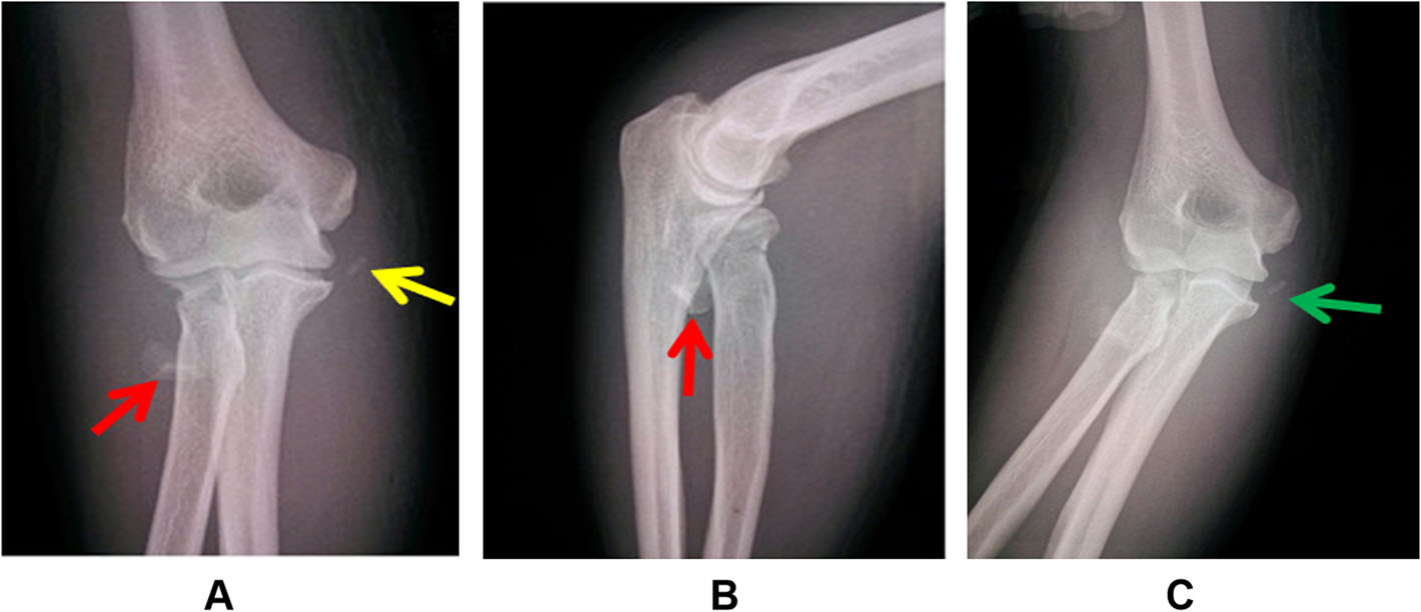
Anteroposterior (a) and lateral (b) radiographs of the injured elbow. The fracture fragment of the radial head (red arrow) is remarkably displaced. A free bone fragment is found on the medial side of the elbow (yellow arrow). The valgus stress radiograph of the injured elbow (c) demonstrates medial joint space widening (green arrow)
